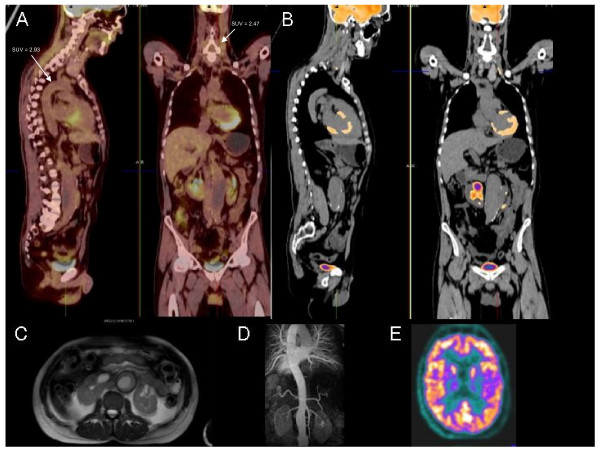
Follow-up 18F-FDG PET-CT study revealed reduced wall enhancement under immunosuppressive therapy. (A), (B) Resolution of fluorodeoxyglucose uptake after immunosuppressive treatment. (C), (D) T2-weighted images and magnetic resonance angiography appear normal except for infrarenal aorta aneurysm. (E) Improvement of metabolism in the left temporal region.
